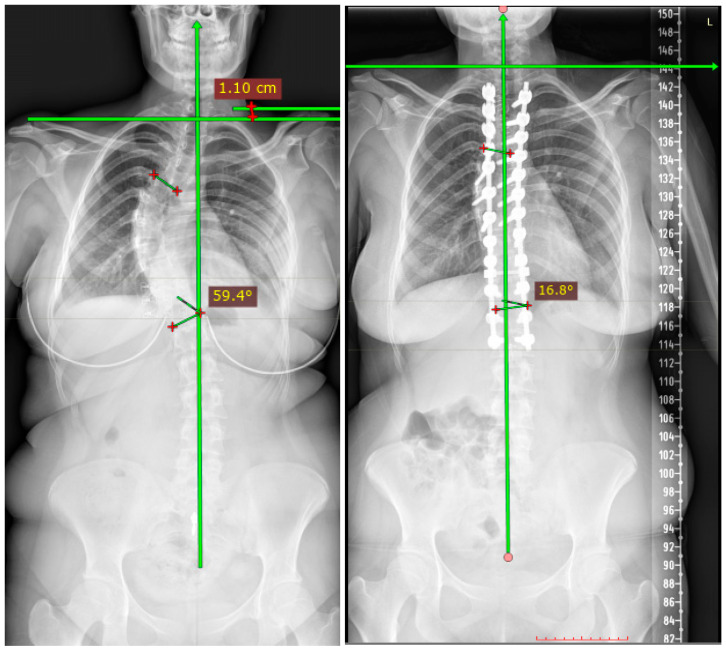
Representative preoperative and postoperative standing posteroanterior radiographs demonstrating coronal curve correction and proximal balance in a patient fused to T2 as the upper instrumented vertebra. Changes are shown longitudinally within the same patient. “L” indicates the left side of the patient, and “+” marks the reference points used for angular measurement.
